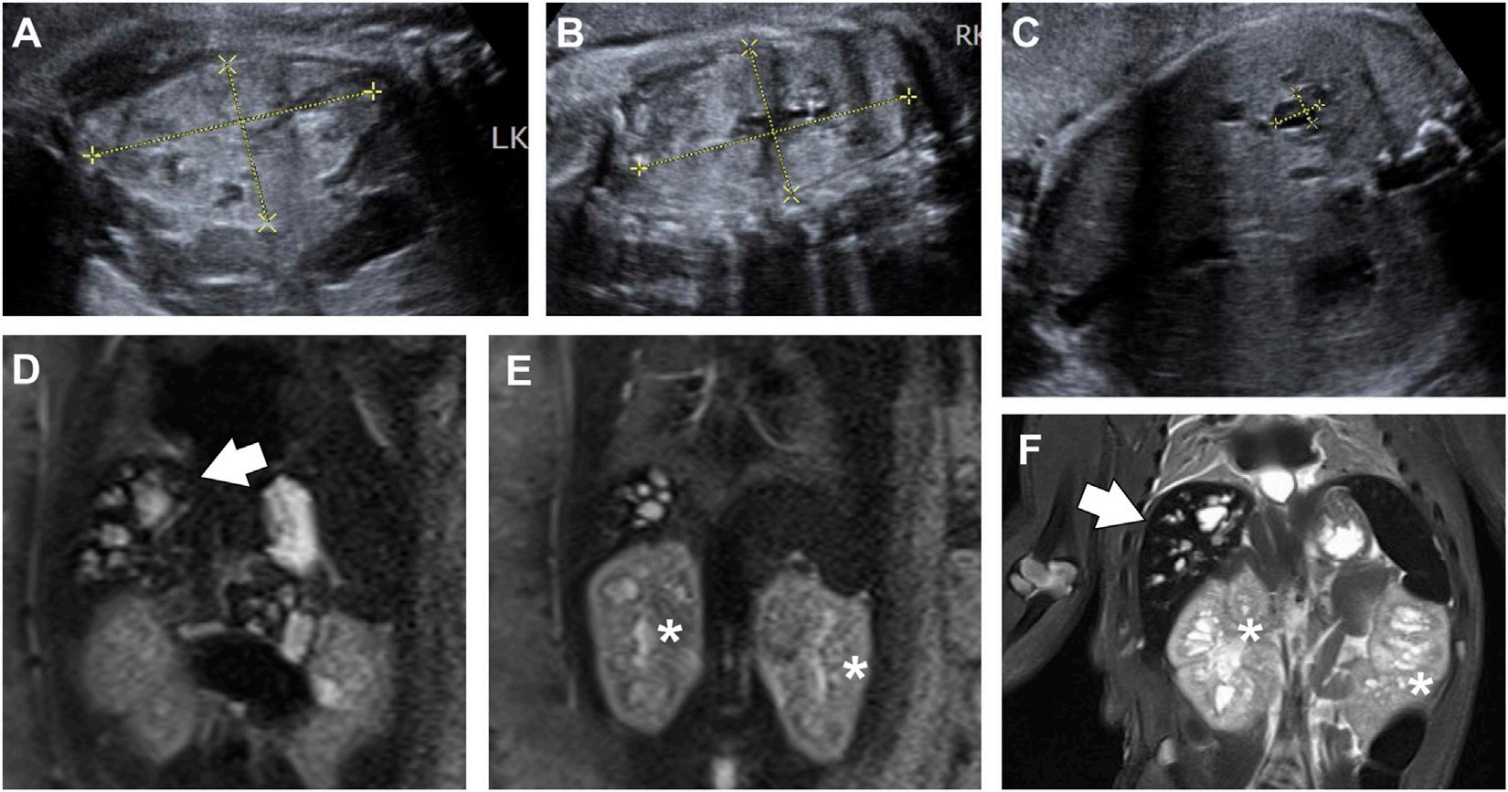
View of fetal abdomen before (A–E) and after birth (F). (A-C) Ultrasound images of fetal kidney (A,B) and liver (C) at 39+2 weeks of gestation. Both kidneys ((A,B); left, 6.28 cm × 3.57 cm; right, 6.67 cm × 3.65 cm) were enlarged and echogenic, with no corticomedullary differentiation. The right lobe of the liver (C) showed diffuse cystic structures, one of which was 1.03 cm × 0.74 cm in size. (D,E) T2-weighted MRI image of fetal abdomen at 39+6 weeks. The fetal liver (D, arrows) was hyperintense on T2-weighted sequences, showing multiple cystic and tubular structures. Both kidneys [(E), asterisks] were enlarged and heterogeneous, with numerous micro cysts. (F) T2-weighted MRI image of the baby, showing dilated intrahepatic biliary ducts (arrows), and slightly enlarged kidneys (asterisks) with cystic structures.
